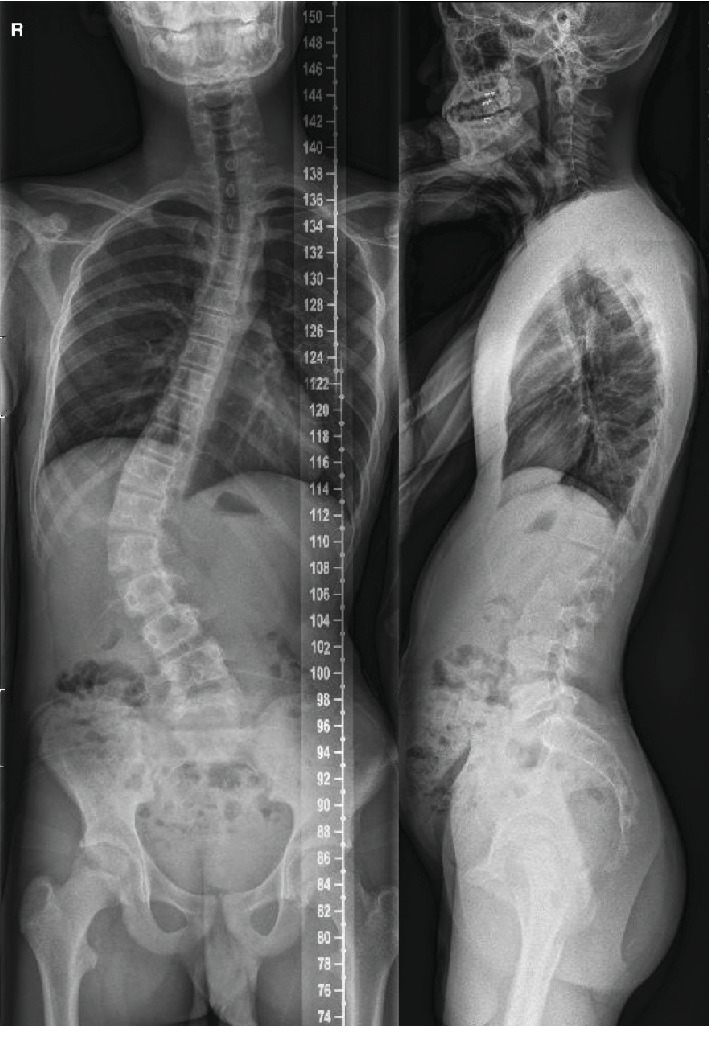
Whole spine plain radiograph. AP and lateral view when surgery was decided for the patient, at December 28^th^, 2015.
